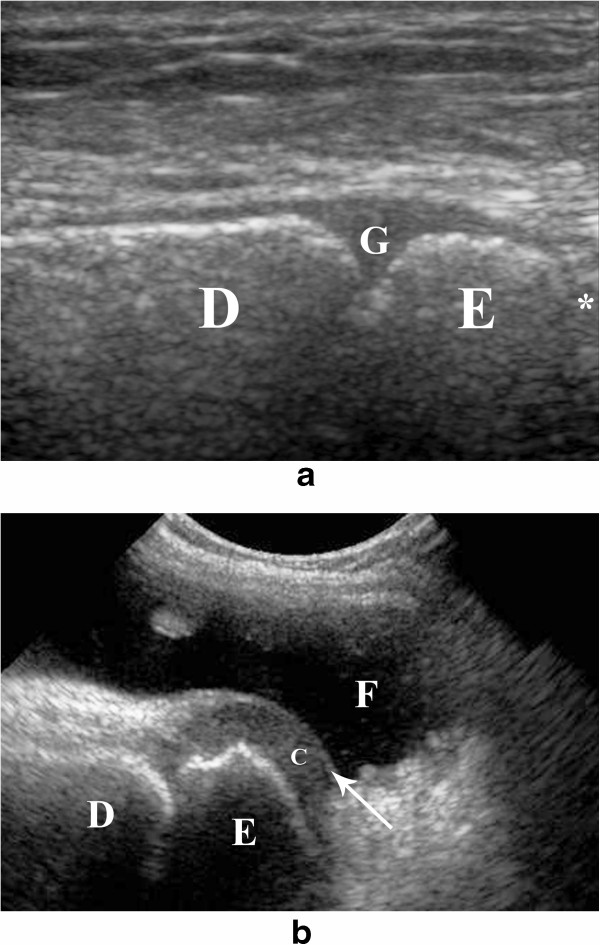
Sonographic appearance of the developing bone. a. Longitudinal sonographic view of the distal tibia showing the distal growth plate (G) as a hypo-echoic area, in between the diaphysis (D) and epiphysis (E). The growth plate should be differentiated from the joint space (*), which is located distal to the epiphysis. b. Longitudinal sonographic view of the knee joint in a 5 years old girl. Distal femoral epiphysis (E) is visualized distal to the diaphysis (D). The epiphysis shows peripheral non-ossified cartilage (c). The articular cartilage is seen as a superficial echogenic line overlying the cartilage (arrow). The differentiation of the joint components is enhanced by the acoustic window provided by a significant joint effusion (F).
